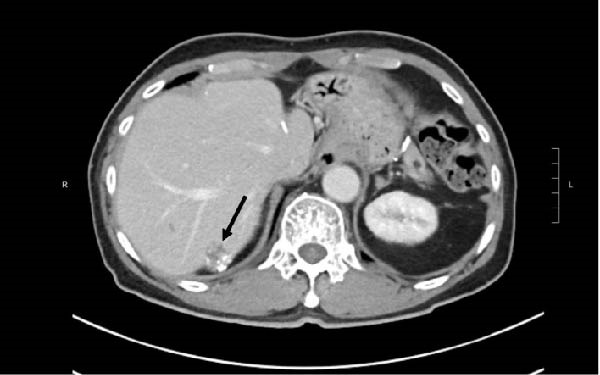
CT showing a recurrent seg 6/7 tumor (arrow).
